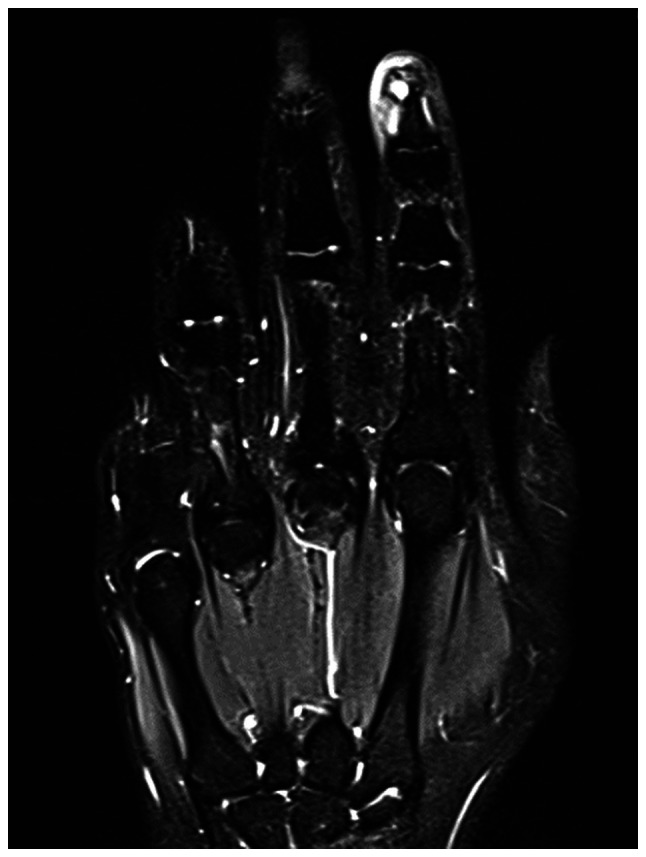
Coronal T2-weighted fat suppression showing high signal intensity.
